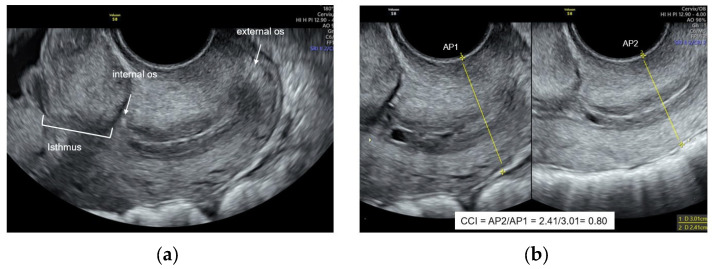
Cervical measurements. (a) Ultrasound image characteristics for first-trimester cervical length measurement. (b) Anteroposterior diameter before pressure (AP1) and anteroposterior diameter after probe pressure (AP2) to obtain the cervical consistency index (CCI).
